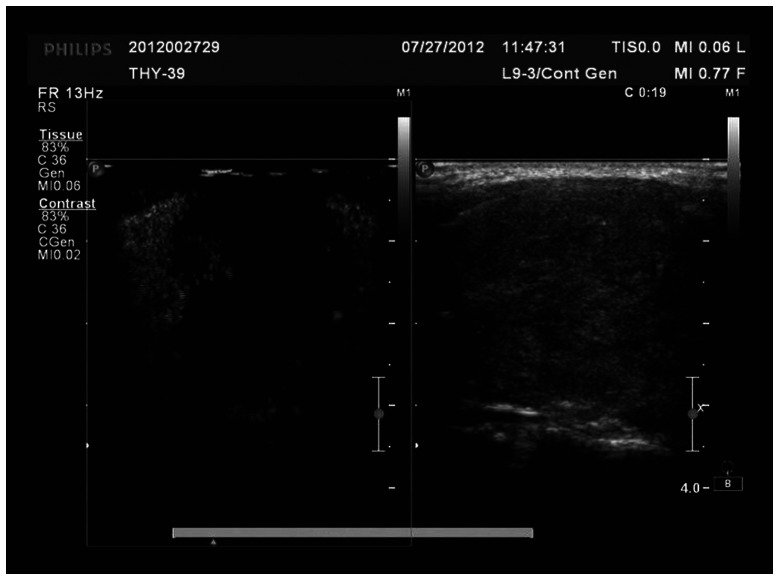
Irregular weak concentric ring enhancement by CEUS (pathologically diagnosed as PTC). CEUS, contrast-enhanced ultrasonography; PTC, papillary thyroid carcinoma.
